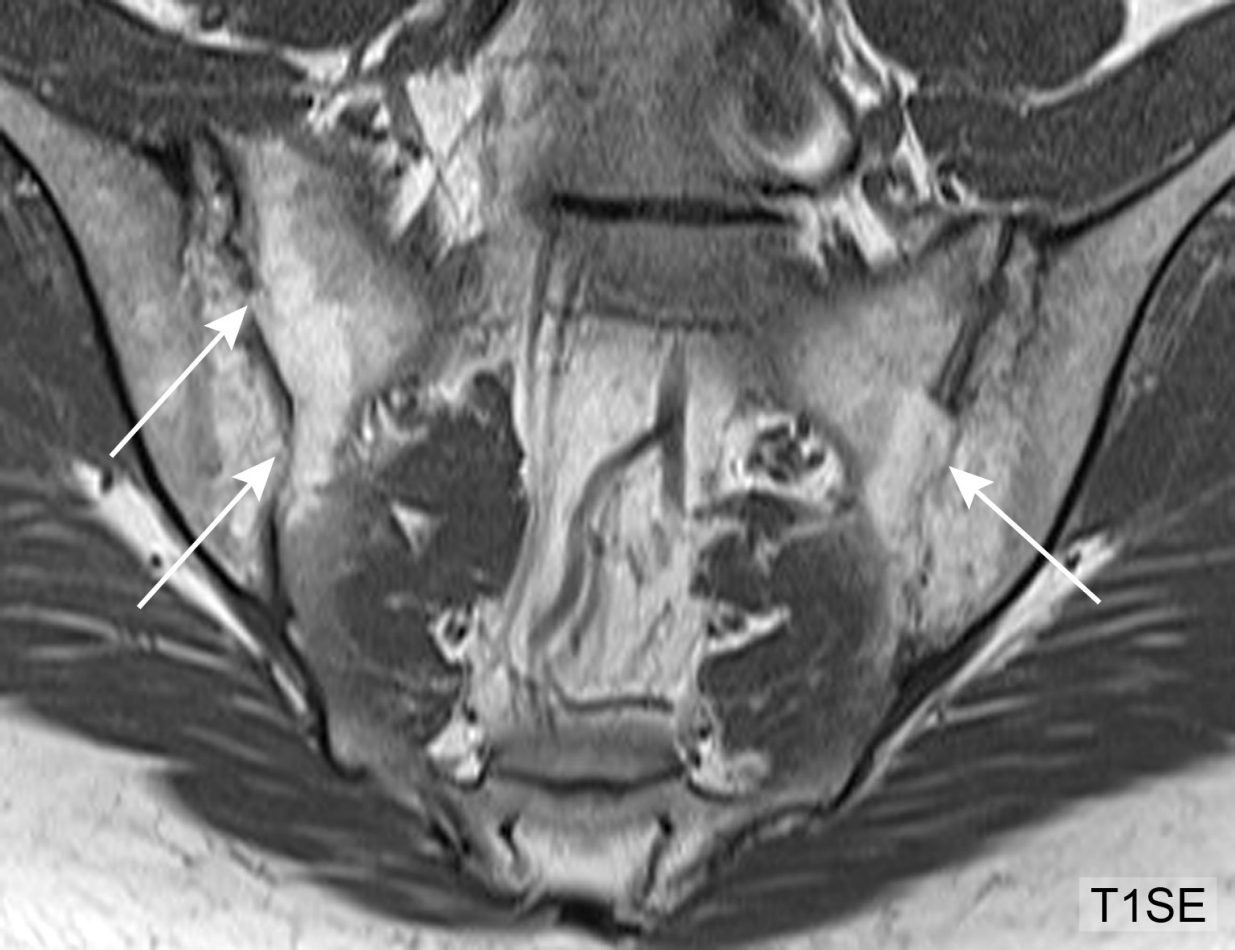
Backfill, follow-up MRI after five years (T1SE sequence). Five years later, the same patient as in Figure 3 shows marked progression of structural lesions in both SIJ with ankylosis in previous areas of backfill (arrows). MRI, magnetic resonance imaging; SIJ, sacroiliac joint; T1SE, T1-weighted spin echo sequence.
